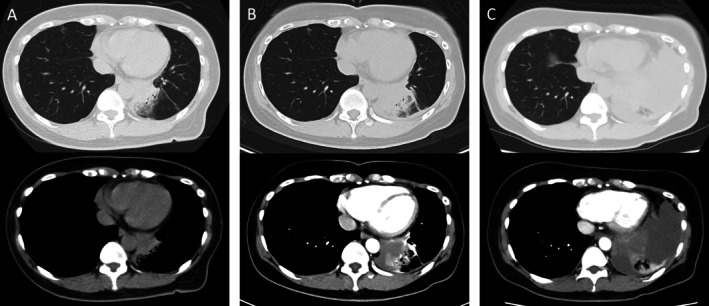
Computed tomography (CT) image. Initial CT (A) shows consolidation in the left lower lobe with surrounding ground‐glass opacity. Three days later, follow‐up CT (B) demonstrates an irregularly marginated nodule within the consolidation and a newly appeared small left pleural effusion. One week later, CT (C) shows marked enlargement of the irregular mass along with a large amount of high‐attenuation pleural effusion, consistent with hemothorax.
